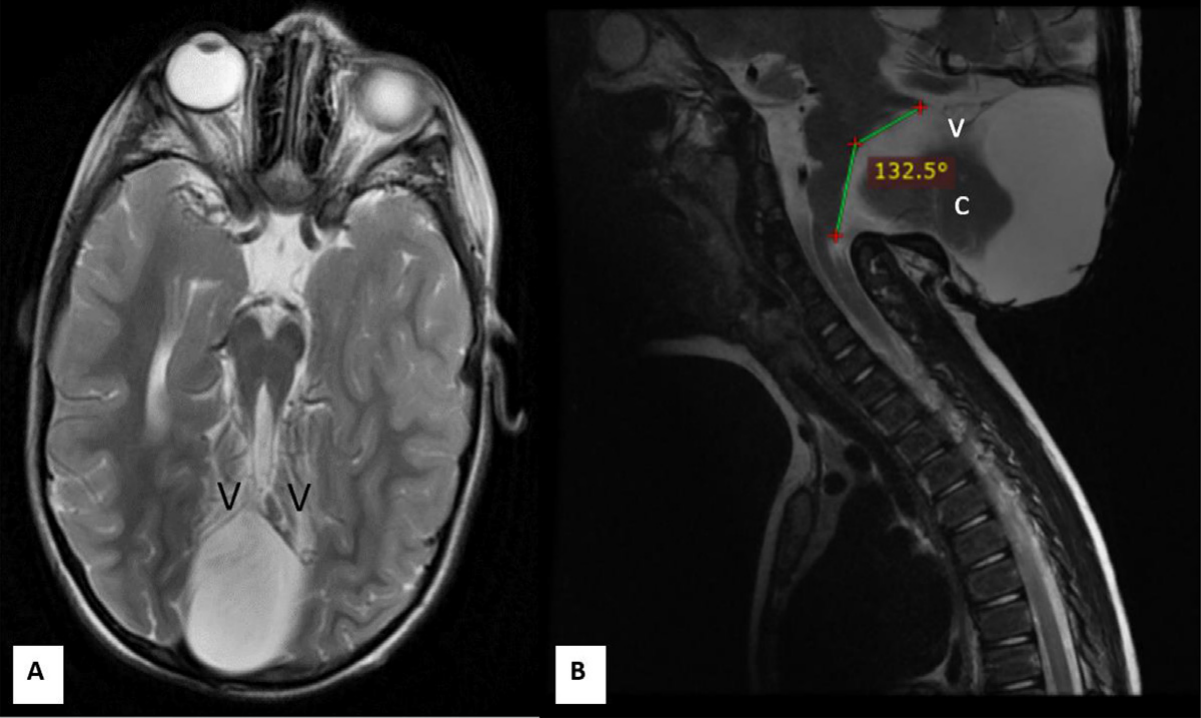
Joubert Plus Syndrome. Axial (A) and sagittal (B) T2-weighted MRI images. The tegmento-vermian angle is markedly elevated, measuring approximately 132.5°, consistent with vermian hypogenesis. The caudal and posterior portions of the vermis are absent, with only a rudimentary superior remnant visible (indicated by “v”). Due to the interdigitating midline configuration, some portion of the cerebellar hemisphere appears in the midline (labeled “c”).
